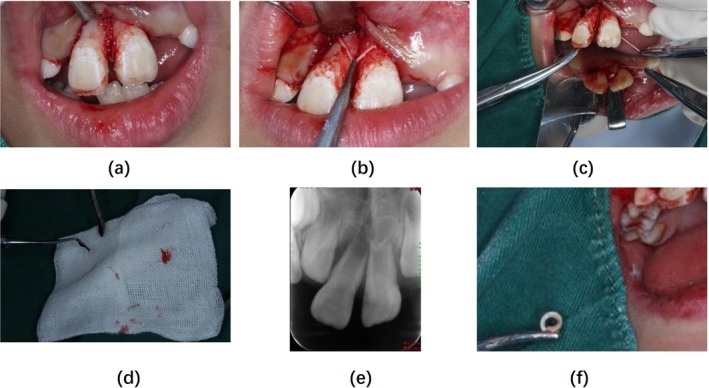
Clinical views during the flap surgery and postoperative radiograph. (a) Surgical site exposed after flap reflection; (b, c) elastic band visualized following flap elevation; (d) resin‐like fragments identified during surgery; (e) removal of the elastic band from the subgingival region; (f) postoperative radiograph showed no residual foreign material.
